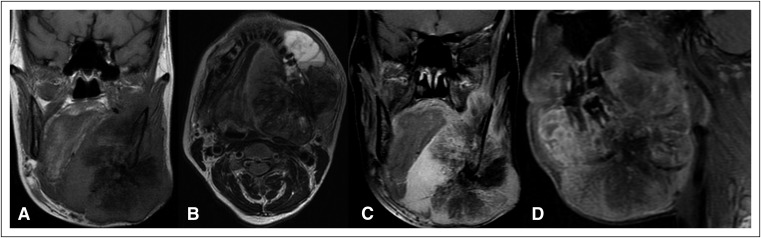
(A) Plain T1-weighted coronal, (B) T2-weighted axial, and (C) postcontrast T1-weighted magnetic resonance images of the neck reveal an expansile lesion in the left hemimandible extending into the submandibular region, floor of mouth, parapharyngeal space, and masticator space. T1- and T2-weighted images show large areas of low intensity with intervening enhancing soft tissue component within the mass giving a sunburst appearance.
